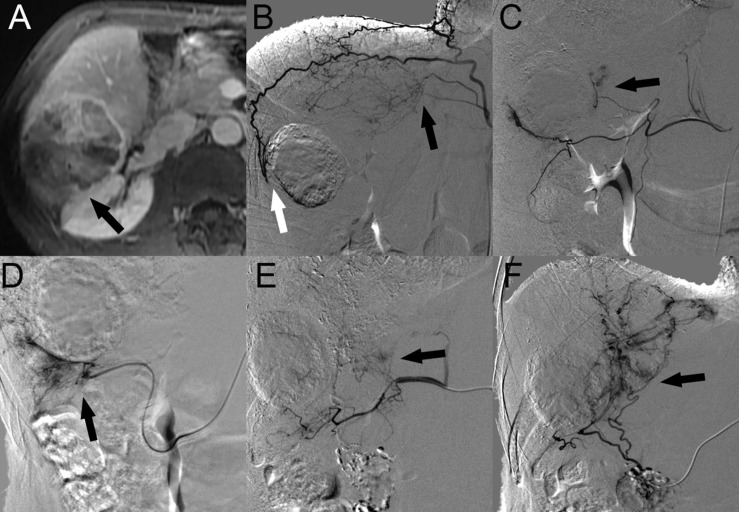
HCC with RAPS invasion in a 51-year-old man. (A) Contrast-enhanced portal phase MR image showed a 7.6-cm-sized tumor (black arrow) in segment 6 of the right lobe of the liver invading the RAPS in the axial plane. (B) Angiographic image at the first recurrence revealed that both the superior branch of the RAA (black arrow) and the posterior branch of the RIPA are involved in the tumor blood supply (white arrow). (C) Angiographic image at the TACE session to the first recurrence revealed that the tumor was also supplied by the middle branch of the RRCA (black arrow). (D) Angiographic image at the second recurrence revealed that the EHCs were supplied by the accessory omental artery (black arrow). (E) After the fourth chemoembolization session, the SA was involved in the tumor blood supply (black arrow). (F) At the last TACE session, the tumor was supplied by the right omental artery (black arrow). Abbreviations: HCC, hepatocellular carcinoma; RAPS, right anterior pararenal space; RIPA, right inferior phrenic artery; RRCA, right renal capsular artery; RAA, right adrenal artery; and SA, supraduodenal artery.
